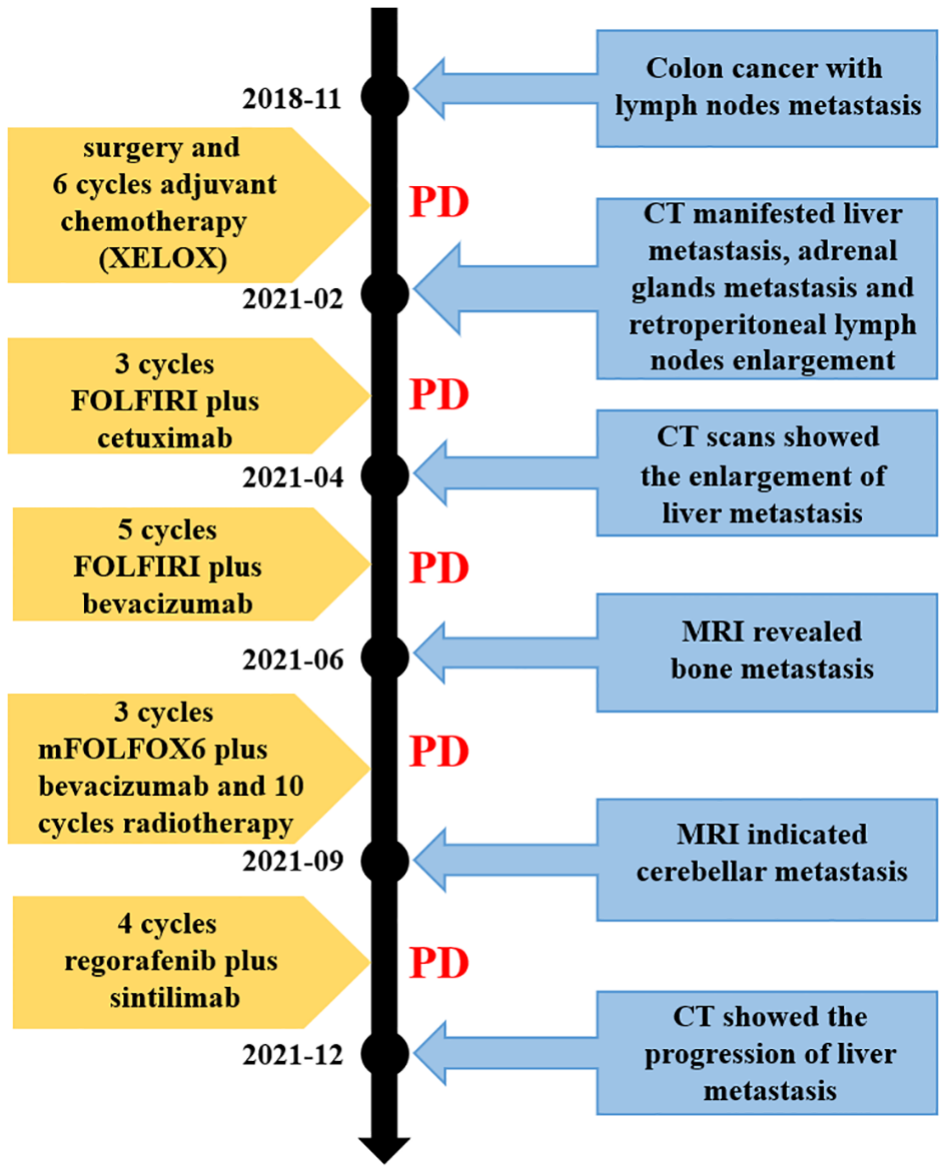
Timeline of the disease evolution. XELOX, chemotherapy regimen containing capecitabine and oxaliplatin; FOLFIRI, chemotherapy regimen containing irinotecan, leucovorin and 5-FU; mFOLFOX6, chemotherapy regimen containing oxaliplatin, leucovorin and 5-FU. CT, computed tomography; MRI, magnetic resonance imaging; PD, progressive disease.
